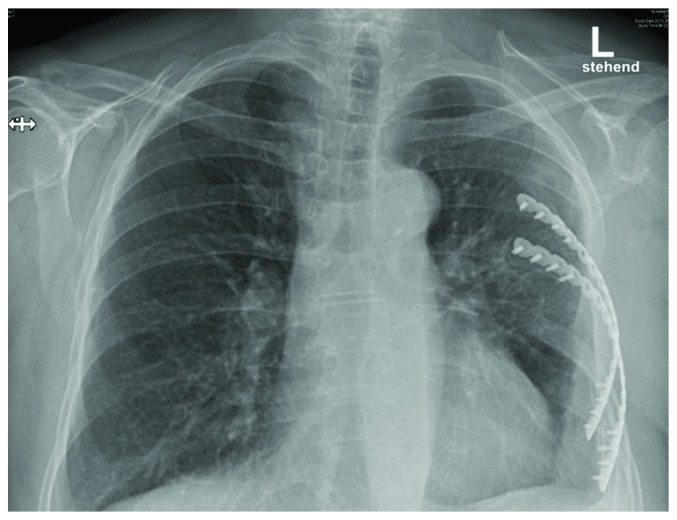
Chest radiography at discharge.
